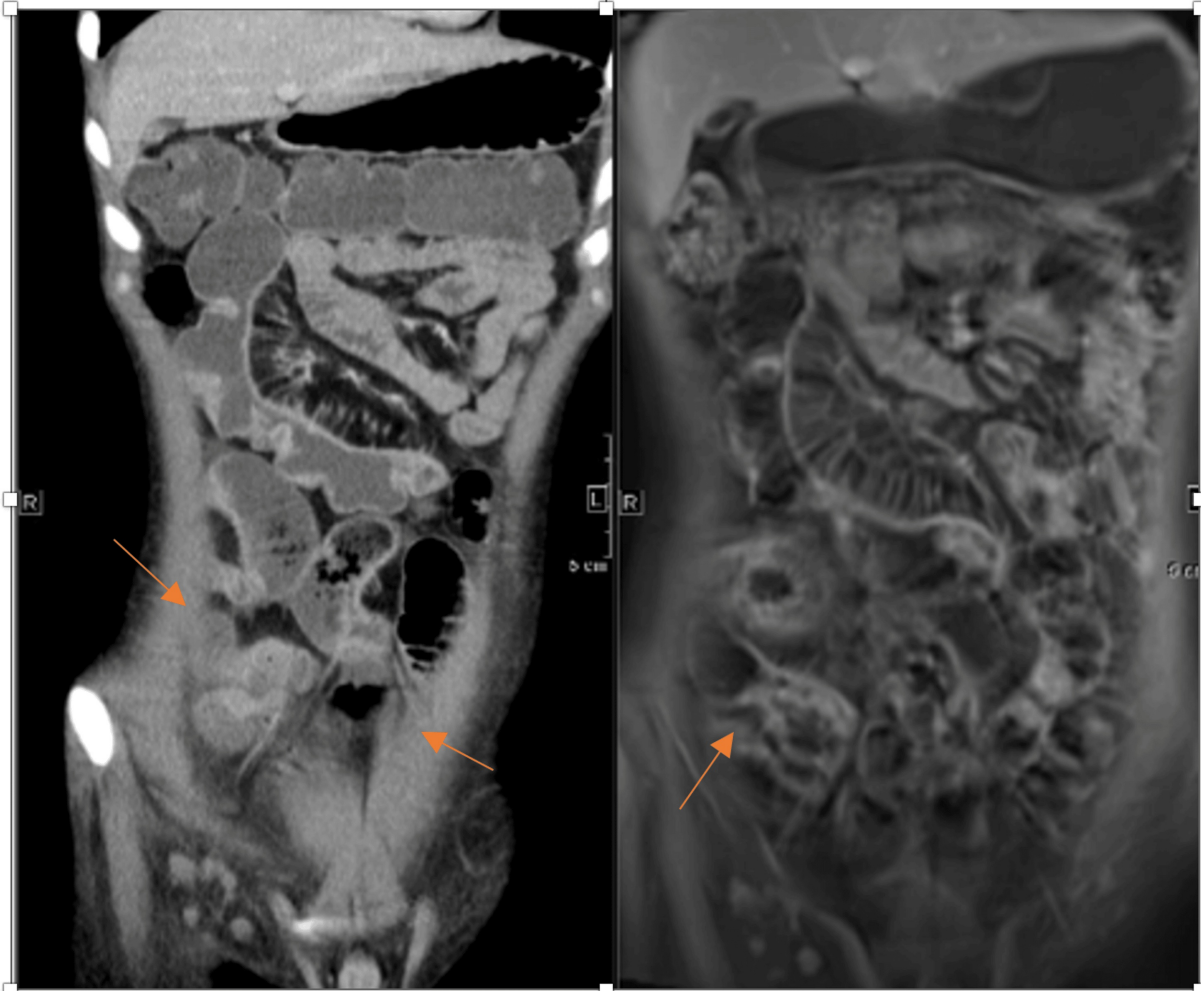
Creeping Fat With Prominent Vasa Recta on a) CTE Coronal Image and B) T1 Post-contrast Coronal Image (Arrow) CTE: Computed Tomography Enterography
